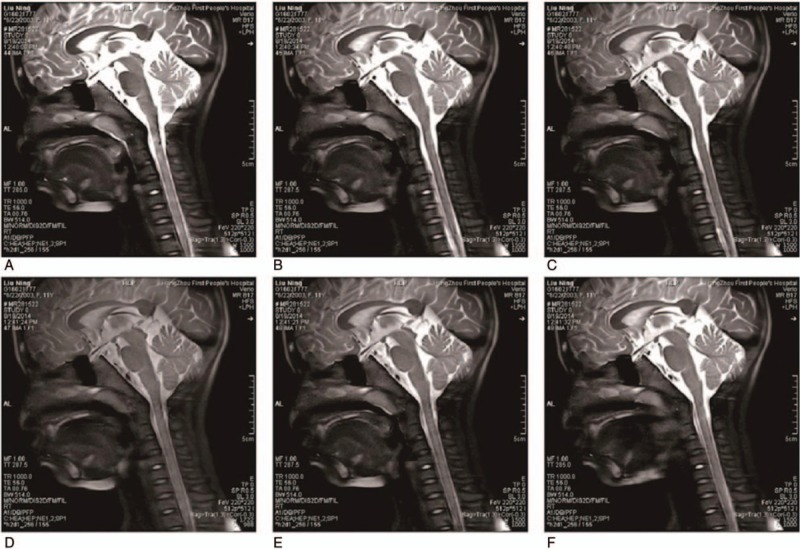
Median sagittal MRI of the upper airway at the 6 respiratory phases. (A–C) Sagittal MR images of the upper airway at 10%, 50%,and 90% of the inspiration phases. (D–F) Sagittal MRI of the upper airway at 10%, 50%, and 90% of the expiration phases.
